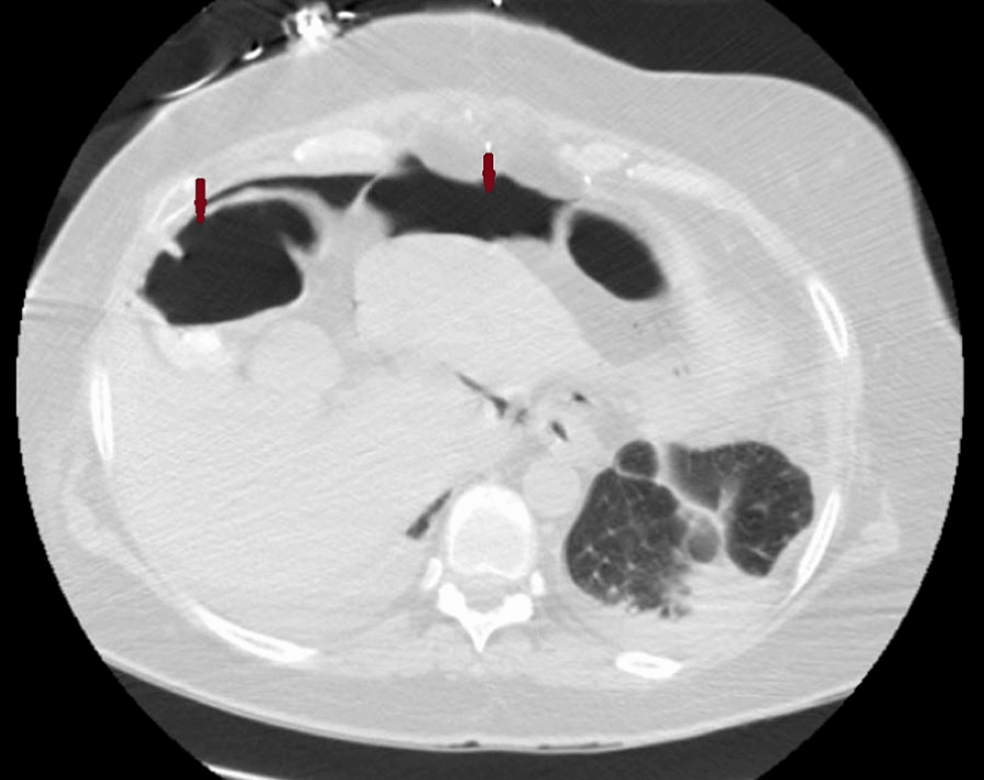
Axial view of computed tomography (CT) chest demonstrating free air in the peritoneal cavity (red arrows)
